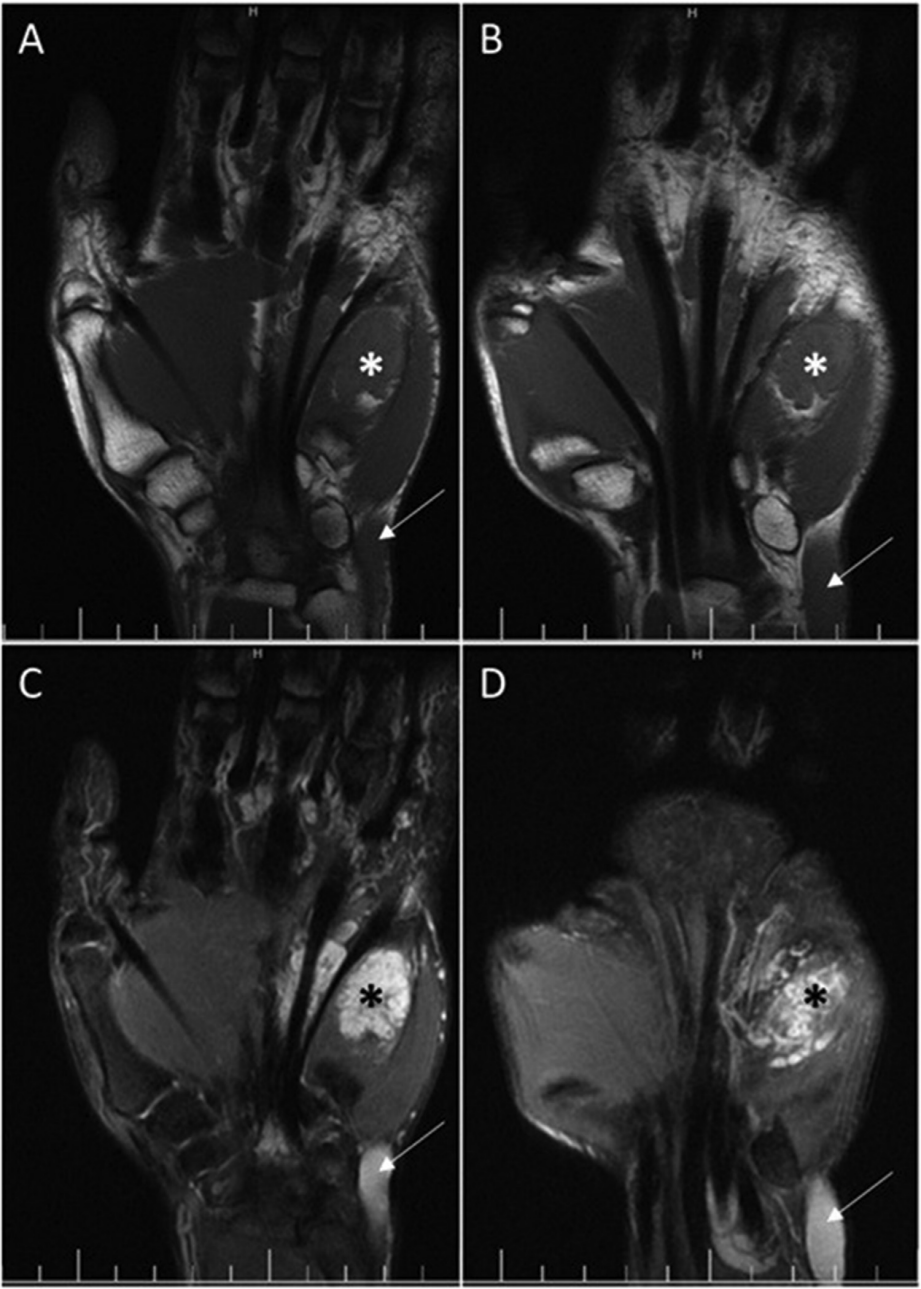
Coronal T1 weighted (A, B) and fat-suppressed T2-weighted (C, D) MR images of the hand and wrist. Soft tissue mass with low signal intensity on T1 weighted images and high signal intensity in T2 weighted images is seen around the flexor tendons (white asterisk). Internal hyposignal areas indicating phleboliths are seen in the palmar region (black asterisk in C and D). Aneurysmal dilatation of the basilic vein is noted (arrow).
